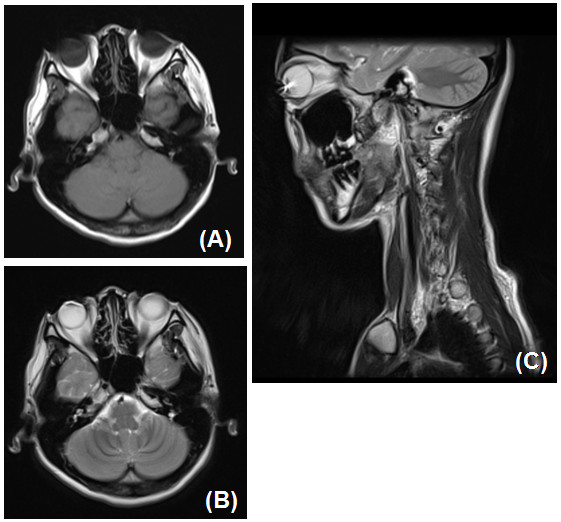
A 28-year-old woman who wore circle contact lenses. MRI showed a susceptibility artifacts in her eyes. A. T1-weighted fast spin-echo axial image: TR/TE, 545/15 (msec). B. T2-weighted fast spin-echo axial image: TR/TE, 4130/83 (msec). C. T2-weighted PROPELLER sagittal image: TR/TE, 3000/83 (msec). TR: repetition time, TE: echo time, PROPELLER: Periodically Rotated Overlapping Parallel Lines with Enhanced Reconstruction.
